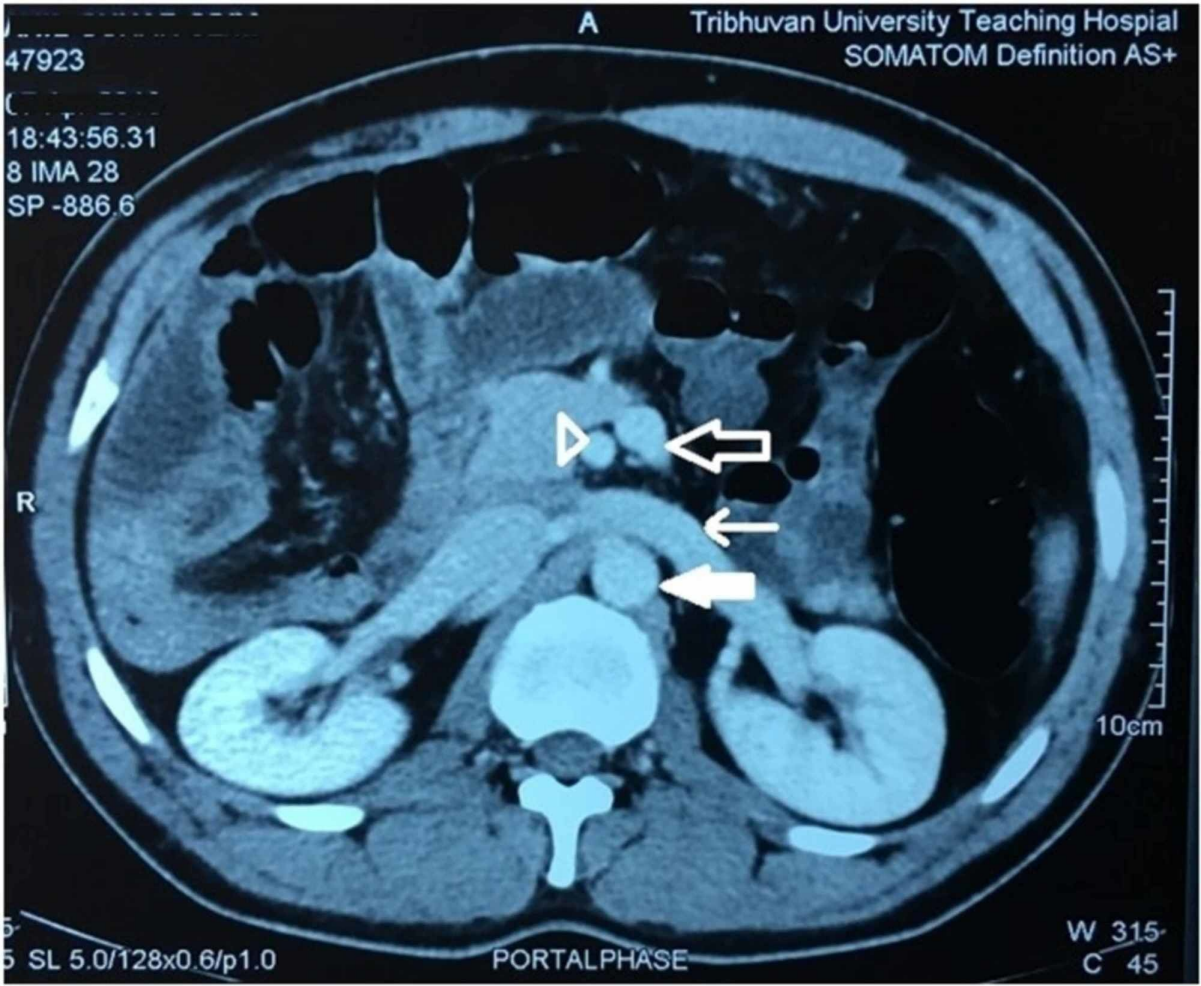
Axial CECT of the abdomen (portal phase) showing the inverse relationship between SMA (arrowhead) and SMV (unfilled block arrow) along with the presence of renal vein (line arrow) and absence of duodenum between SMA and aorta (filled block arrow) CECT, contrast-enhanced computed tomography; SMA, superior mesenteric artery; SMV, superior mesenteric vein
